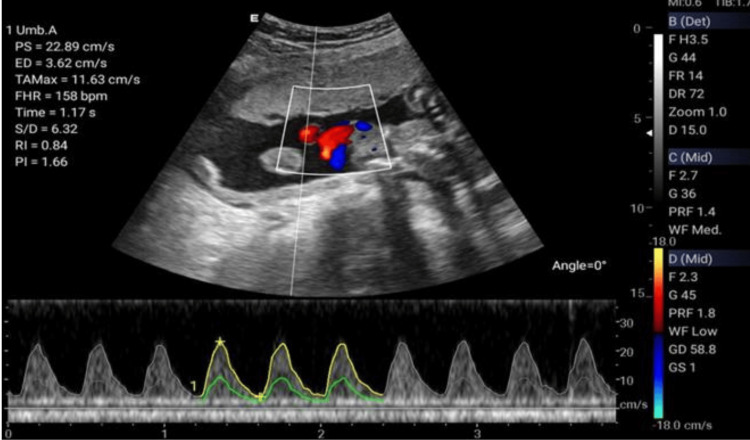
Umbilical artery Doppler.
